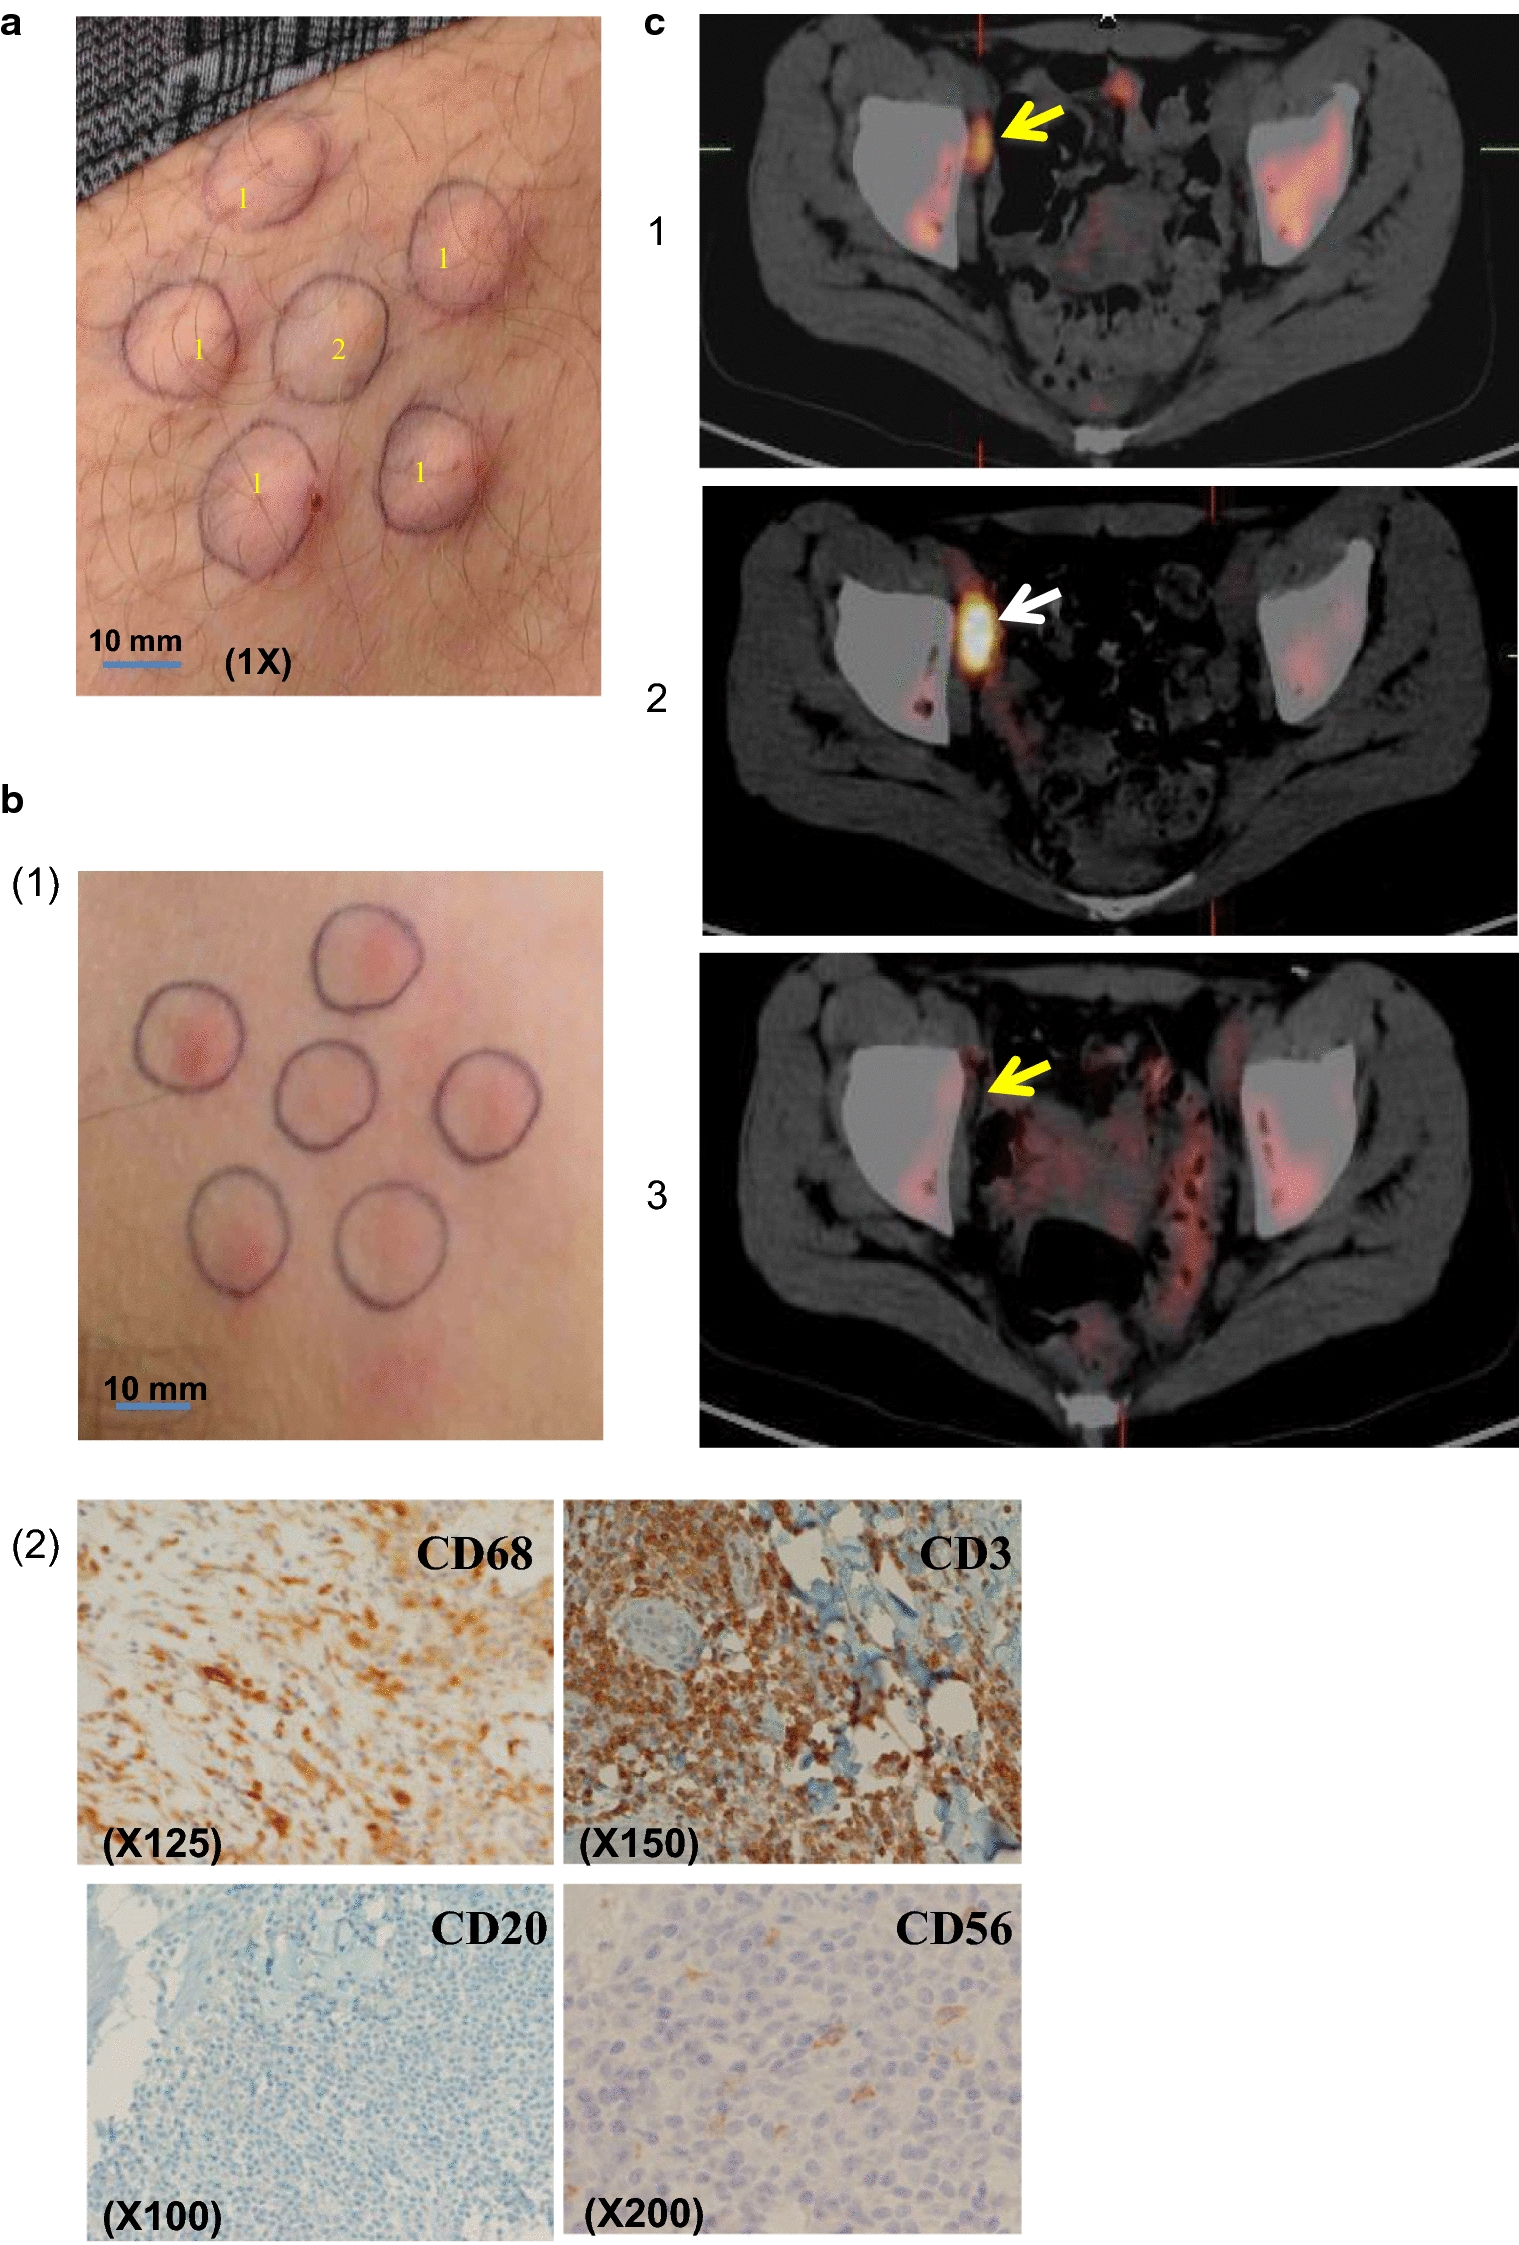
A phase II clinical trial with DC pulsed with tumoral cell lysates from patients having follicular lymphoma. a Intradermal injection of 0.2 mL of DC-pulsed with tumoral lysates (5 × 10^6^ DC/mL) (5 sites) and KLH intradermal injection (100 µg in 0.2 mL) used as a control. Magnification: ×1; b Delayed hypersensitivity after intradermal injections (1) and cutaneous biopsies (2) showing important CD3 positive infiltrate and CD68 positive macrophages; c [18F] fluorodeoxyglucose-positron emission tomography scanning (FDG pet-scan): (1) before vaccination with tumoral lymph node (yellow arrow), (2) 15 days after the vaccination with increased hypermetabolism of the lymph node (white arrow), (3) 2 months after the vaccination with the disappearance of the hypermetabolism (yellow arrow), suggesting an activation of the IECs within the lymph node. 1: injection site with 0.2 mL of DC-pulsed with tumoral lysates; 2: injection site of control; DC: dendritic cells; KLH: Keyhole limpet hemocyanin
